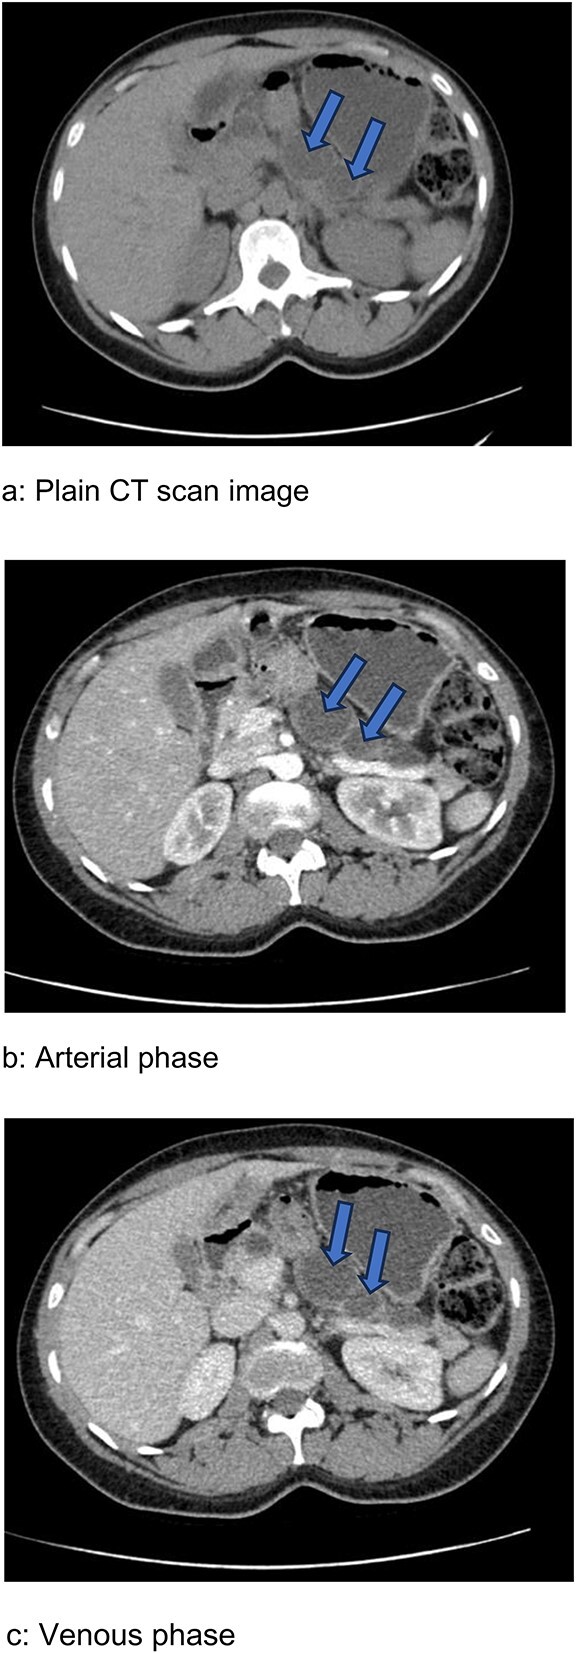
Abdominal CT scan images showing series of cystic dilatations (arrows) of the main pancreatic duct with thinned pancreatic tissue in the body and tail apparent in all three phases. (a) Plain image. (b) Arterial phase. (c) Venous phase.
